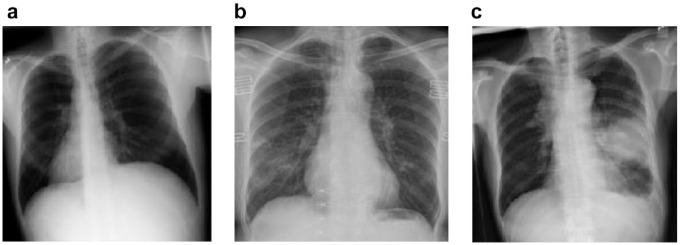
The sample X-ray images used in the experimental analysis of this work: (a) chest X-ray image of normal patient (no-findings), (b) chest X-ray image of coronavirus disease 2019 (COVID-19) patient, and (c) chest X-ray image of pneumonia patient.
